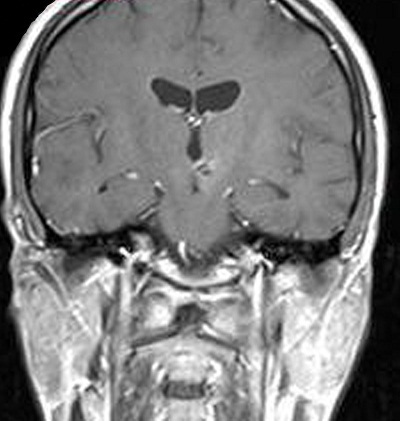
T1-weighted coronal MR image with contrast of the same patient
